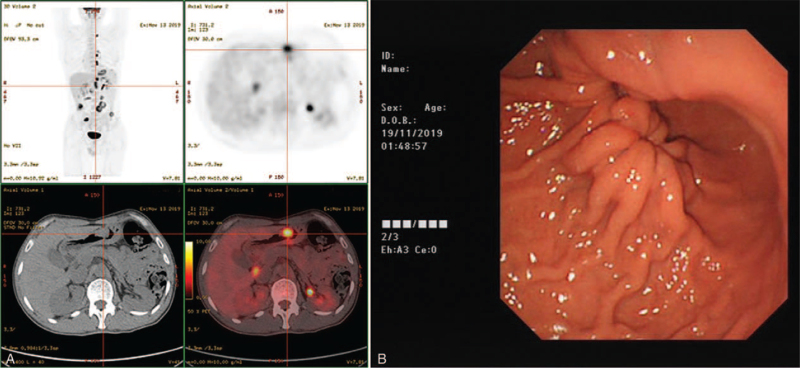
Gastric mucosa in the gastric corpus observed in gastroscopy (B) imaged as a FDG-avid mass (A) in 18F-FDG PET/CT.
